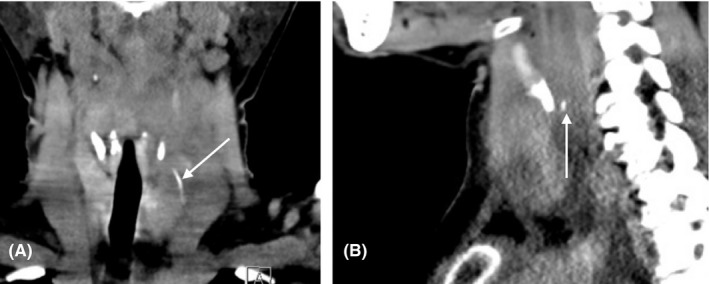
Computed tomography images. A, Coronal image of the fish bone surrounded by an abscess collection. B, Sagittal image of the fish bone. Arrows showing the fish bone
